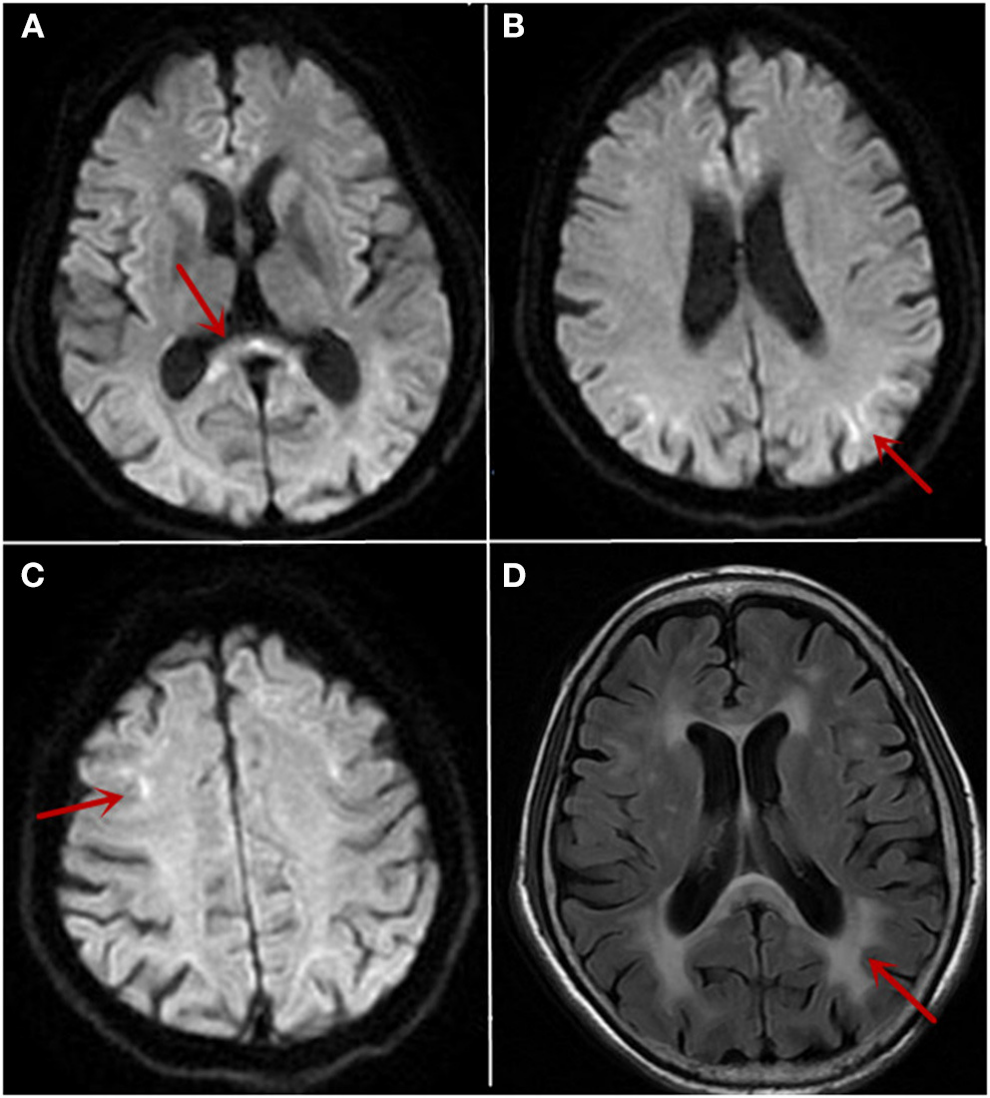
The cranial MRI findings on 10/26/2019 and 12/12/2019. MRI showed high intensity signal in the corpus callosum (A, arrow) and along the corticomedullary junction on diffusion-weighted image (B,C, arrow). White matter hyperintensity on fluid-attenuated inversion recovery images (D, arrow) was also noticed.
